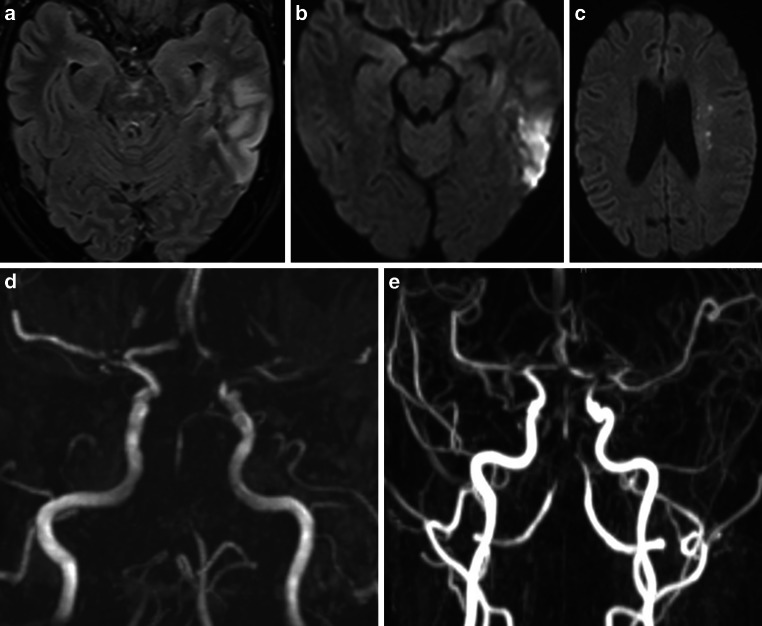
MRI of a patient with severe LNB-associated cerebral vasculitis on day 11 after clinical deterioration. FLAIR image (axial view; a) and DWI images (axial views; b, c) showed progressed left temporal lobe infarction and new ischemic lesions in the deep white matter (watershed zone, hemodynamic infarcts). MIP of the TOF (d) and contrast-enhanced MR angiography (e) demonstrated worsening of the known intracranial stenoses
